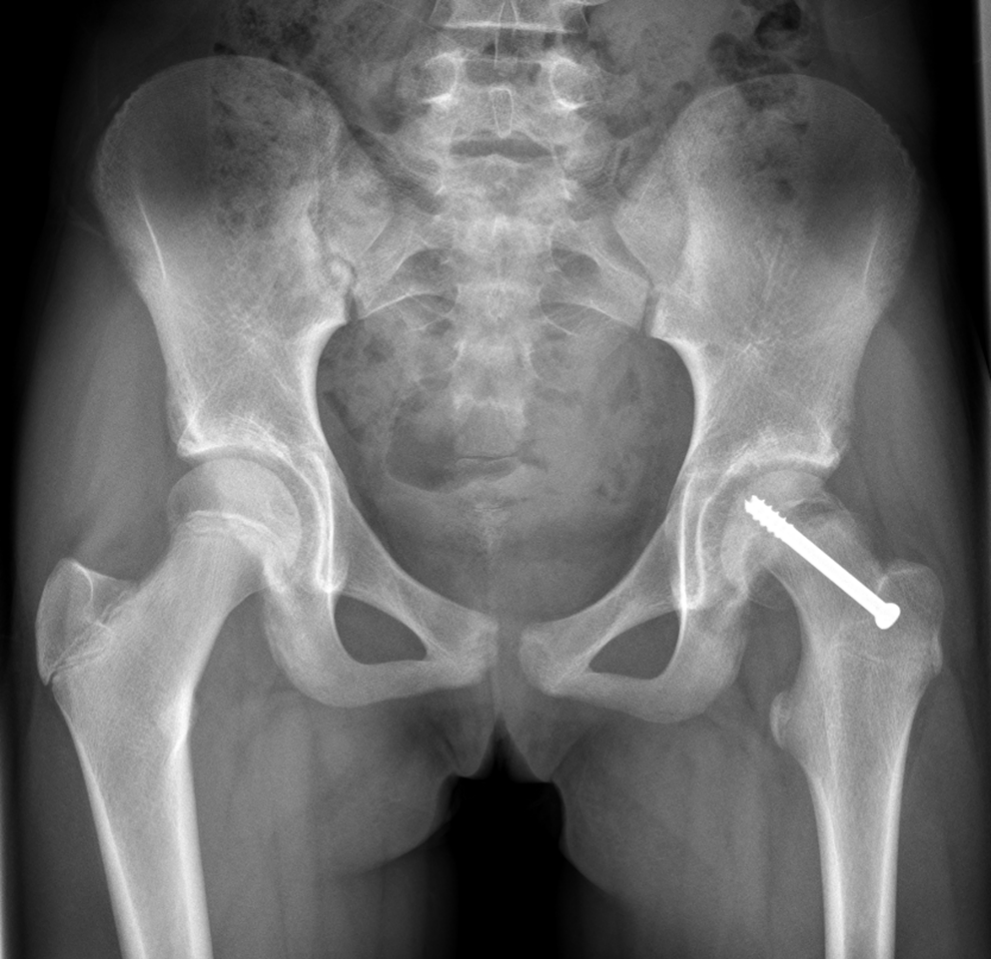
X-ray of a patient with on the left of the image the healthy hip and on the right the affected hip with a pin in-situ
